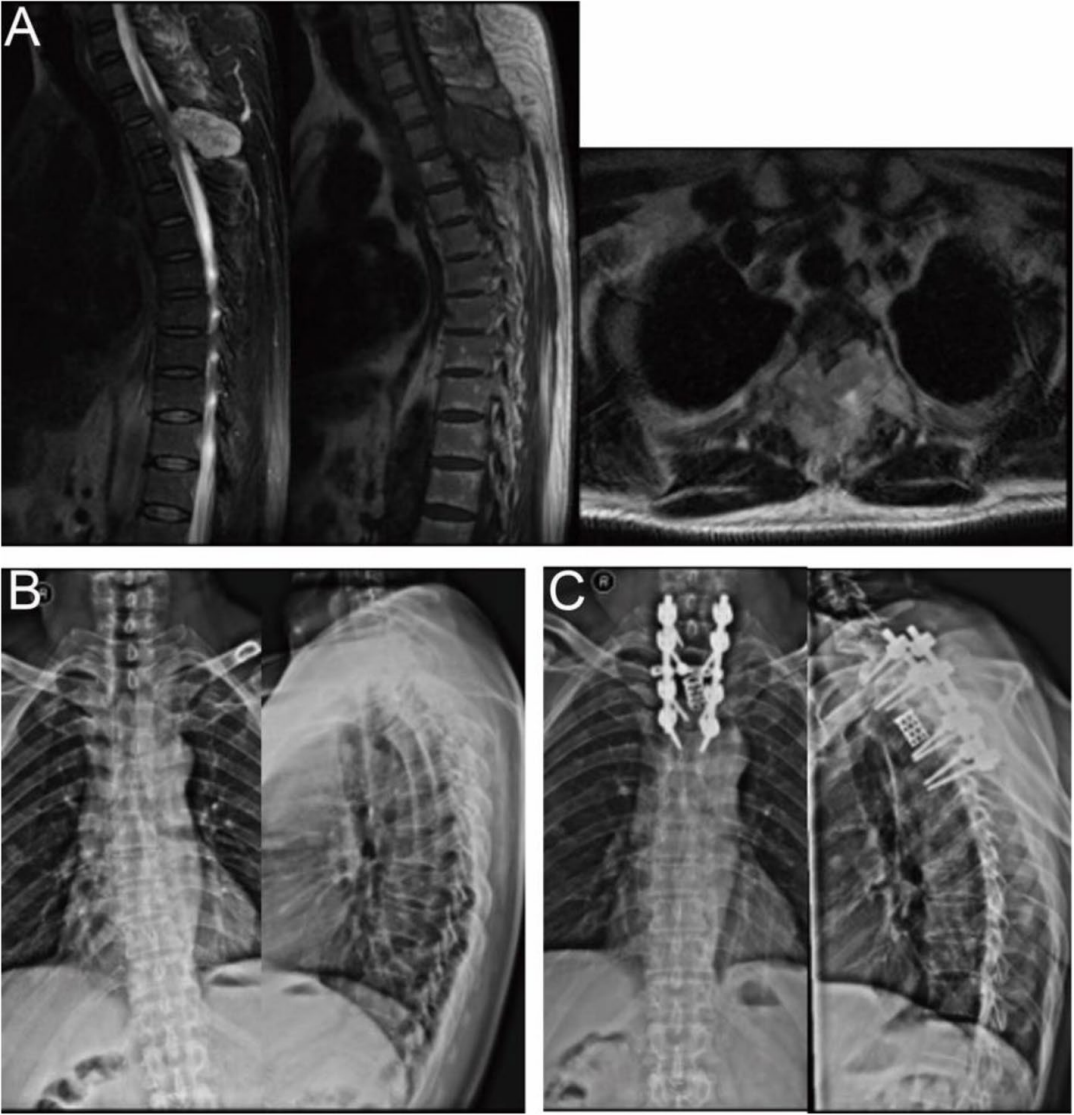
A 57-year-old man with spine metastases of renal cell carcinoma. The patient underwent preoperative arterial embolism and posterior marginal excision (piece-meal). The total blood loss was 7429 ml. A Preoperative sagittal thoracic vertebral MRI showed vertebral collapse at T3. Preoperative transversal MRI showed an extraosseous tumor mass at T3; B Preoperative anteroposterior thoracic vertebral radiography; C Postoperative lateral anteroposterior thoracic vertebral radiography
